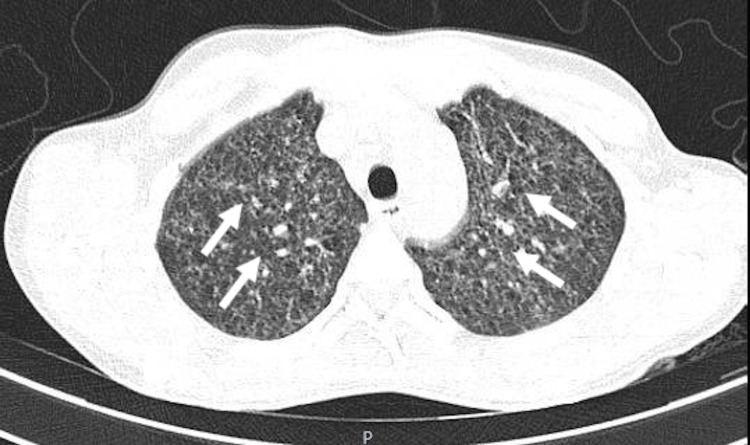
Chest CT image with micronodular pattern suggestive of infection with granulomatous agents.
